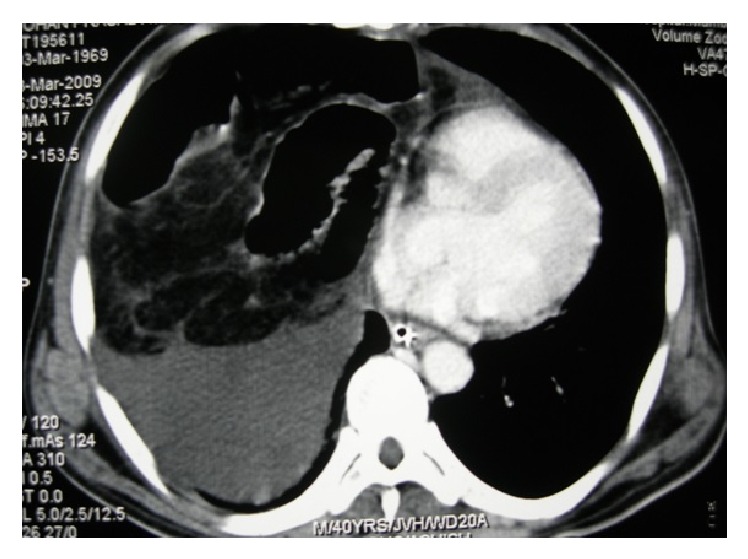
Preoperative CT scan showing thickened small bowel loops in the right hemithorax with air specks in the bowel wall suggesting a strangulated diaphragmatic hernia.
